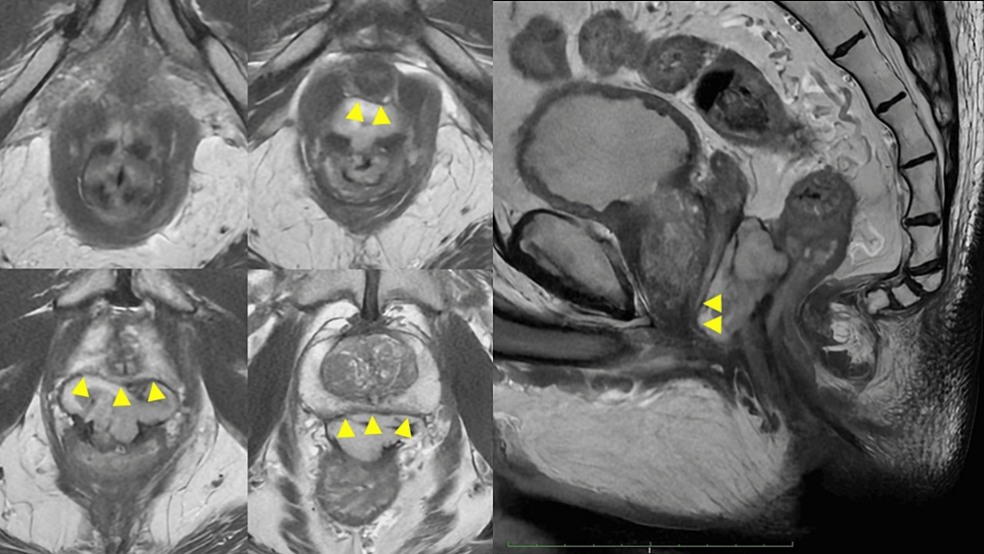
MRI findings after the imatinib treatment The MRI showed that the tumor shrunk to 45 mm, but was still in extensive contact with the prostate and urethra (yellow arrows).
